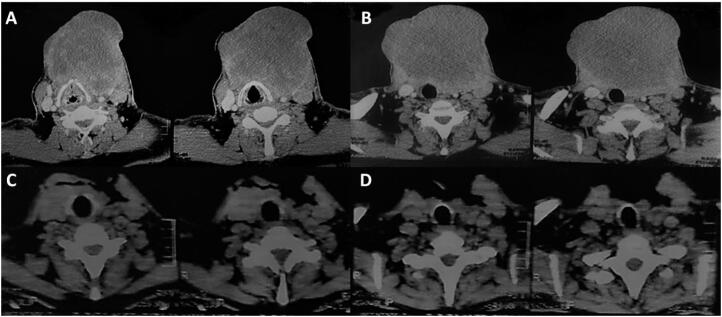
(A and B): Axial view of Computed Tomography (CT) scans of the neck showing the cervical mass before treatment: 120 × 130 mm in the thyroid and left lateral neck. (C and D): Four weeks after treatment, the axial view of CT scans showed a dramatic reduction of the cervical mass around 90%.
